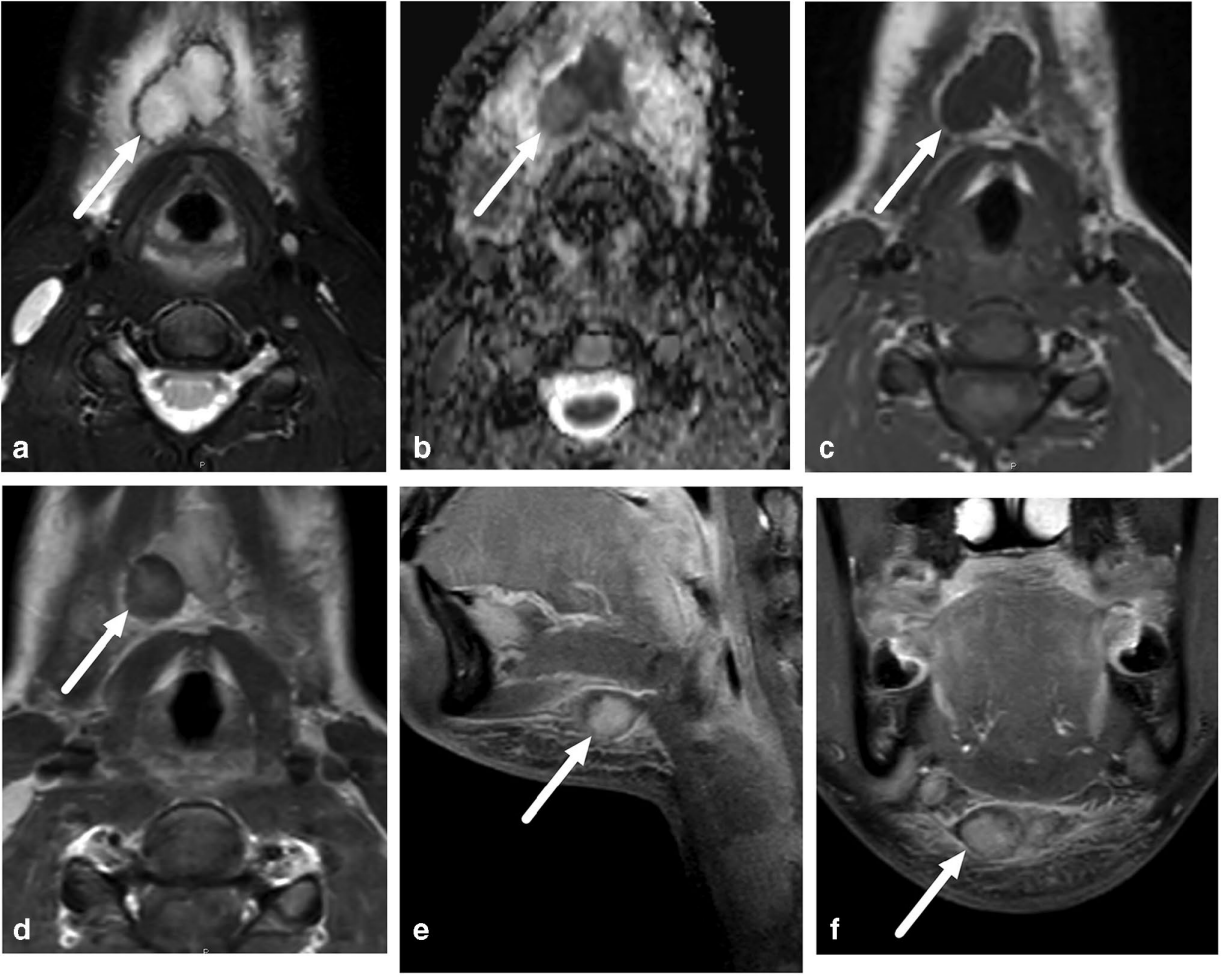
False-positive abscess in a 15-year-old girl with neck swelling. a Axial fat-saturated T2-weighted magnetic resonance imaging (MRI) slices (top left) demonstrates lymphadenitis (*arrow*) and surrounding tissue edema. b Axial apparent diffusion coefficient image shows restricted diffusion in the lesion (*arrow*). c, d Axial T1-weighted images before (c) and after (d) contrast shows faint partial contrast enhancement compared with pre-contrast image (*arrows*). e, f Sagittal (e) and coronal (f) fat-saturated post-contrast T1-weighted images confirm faint enhancement (*arrows*). This finding was interpreted as suppurative lymphadenitis (intranodal abscess). Surgery found necrotic lymphadenitis, but no purulence. This case highlights the difficulties involved in distinguishing necrosis from early abscess
